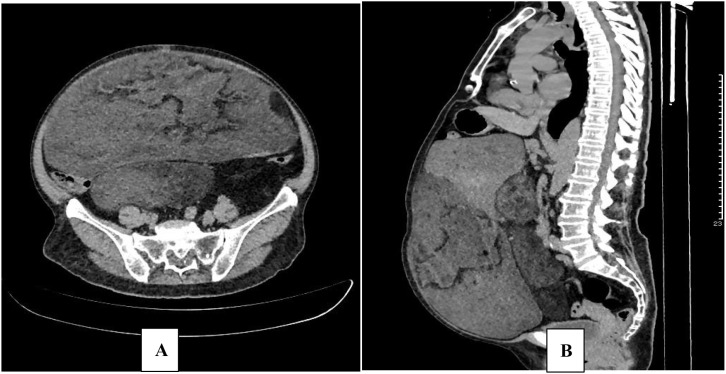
Abdominal CT. Abdominal CT obtained in July 2021 (cycle 16 of eribulin therapy). Stable disease (SD) confirmed by imaging data. Axial (A); Sagittal (B).
